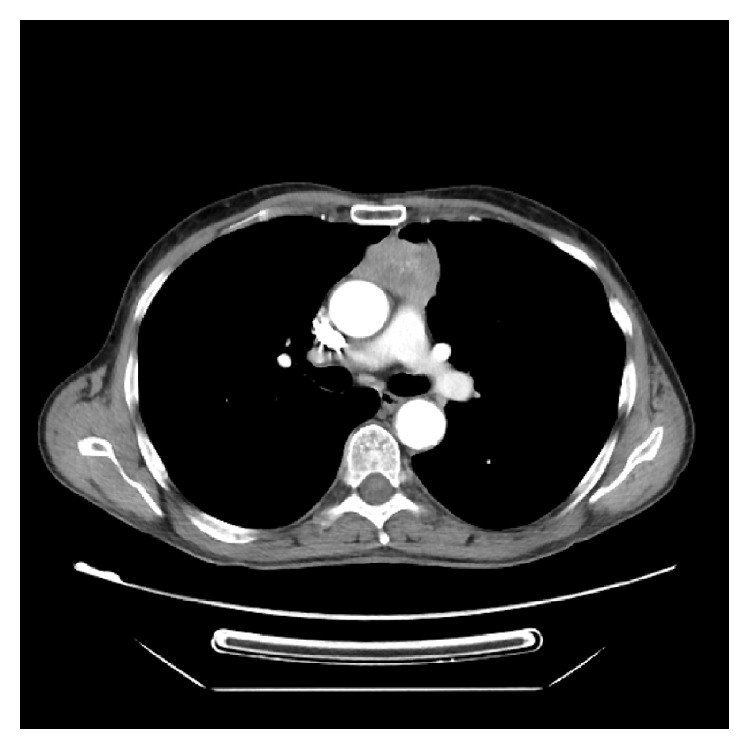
CT scan cross section: anterior mediastinal mass, upper quadrant, with irregular shape, heterogenous iodophilia; it has small areas of necrosis; axial dimension approx 49/29 mm and 50 mm craniocaudal; it is situated between the ascendant aorta and the trunk of the pulmonary artery and has lateral relation with the pulmonary parenchyma.
